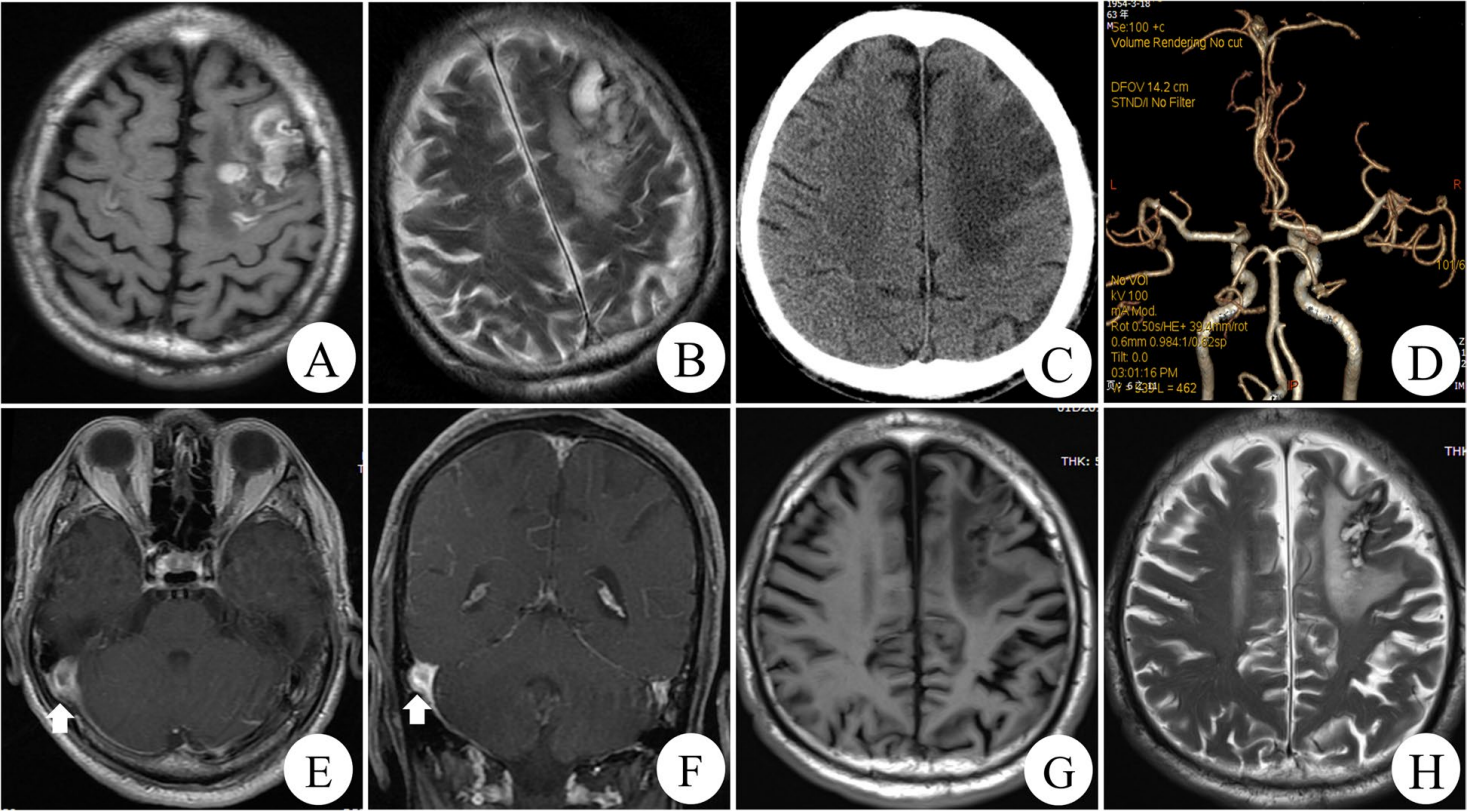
The T1-weighted magnetic resonance images (A), the T2-weighted magnetic resonance images (B) and CT images (C) demonstrate bilateral frontal hemorrhagic infarction. Axial (E) and coronal (F) contrast-enhanced T1-weighted magnetic resonance imaging demonstrate filling defect and a δ sign in the right transverse sinus, sigmoid sinus. The computed tomography angiography in brain is unremarkable except for hypoplasia of the left anterior cerebral artery A1 segment (D). The repeated T1-weighted magnetic resonance images (G) and the T2-weighted magnetic resonance images (H) reveal encephalomalacia in right frontal lobe after 2 years
